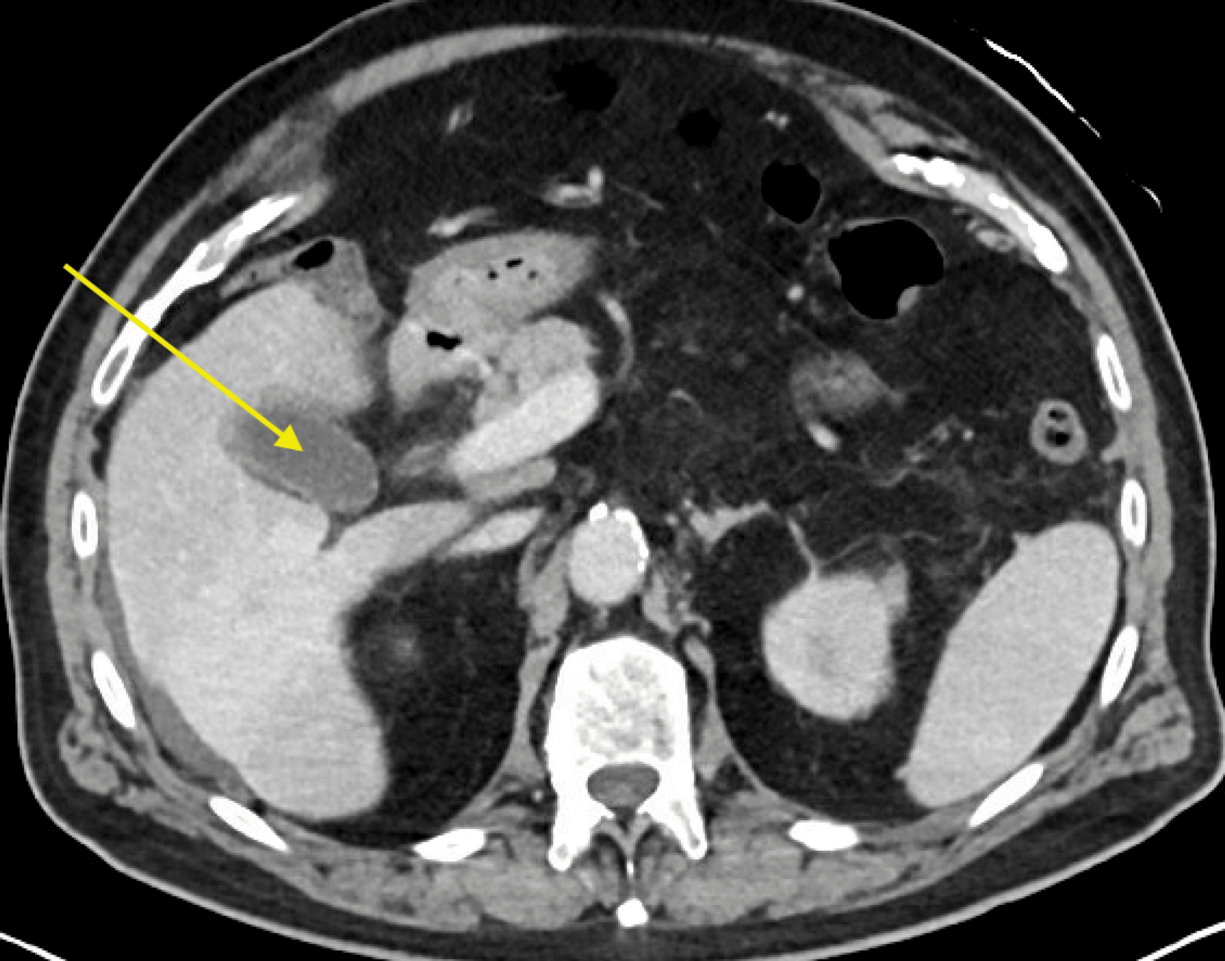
CT abdomen and pelvis showing cholelithiasis with gallbladder wall thickening and pericholecystic fluid. The gallbladder is indicated by the yellow arrow.
